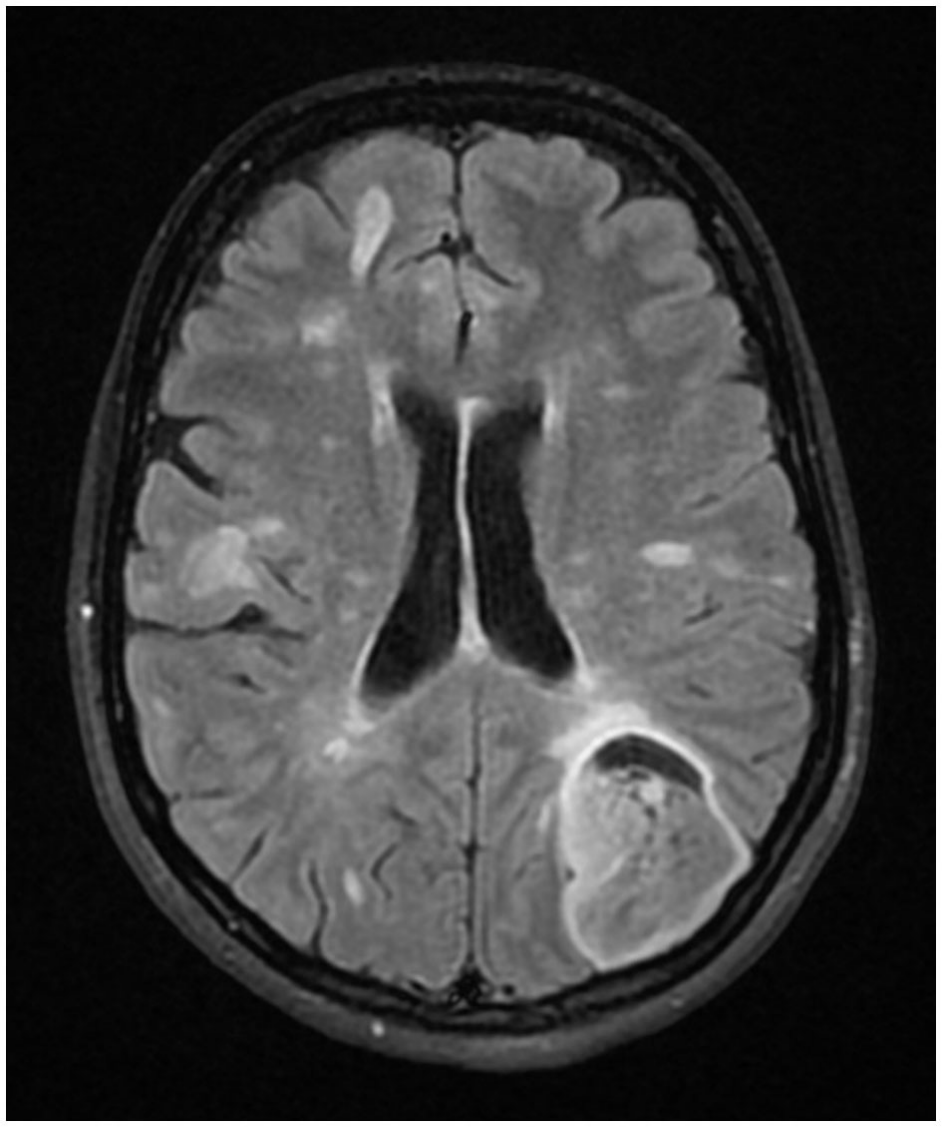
18F-fluorothymidine PET-MRI of the brain.
